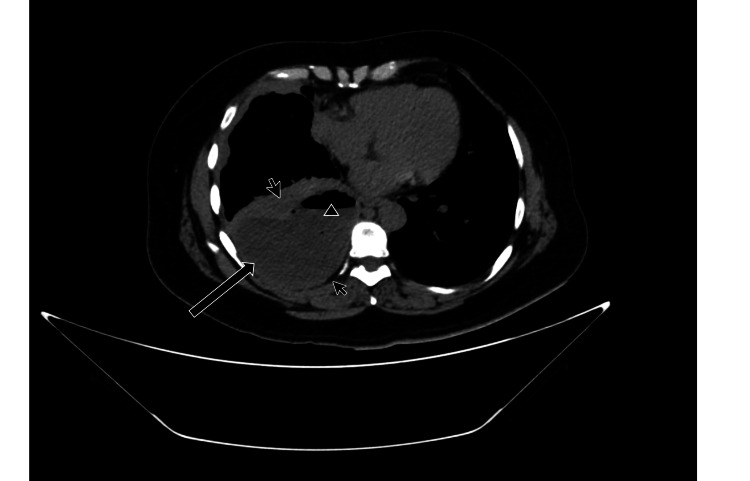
CT pulmonary axial view. Black arrow: Heterogeneous hypodense material in the pleural space. Small arrows: Pleural thickening associated with subpleural fat space preservation. Arrowhead: Hydro-air level.
